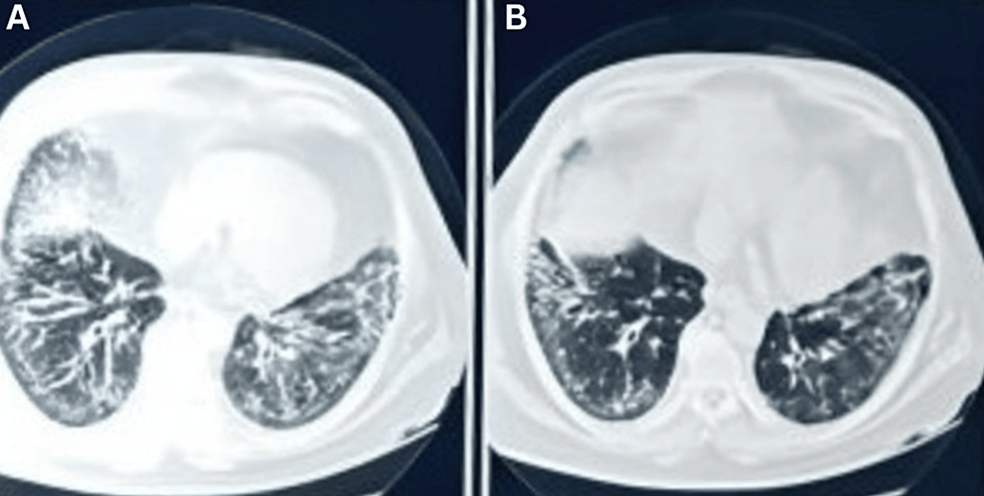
HRCT thorax A: bilateral ground glass opacities with interstitial septal thickening in bilateral lower lobes with subpleural sparing suggestive of interstitial lung disease (non-specific interstitial pneumonia pattern) and bilateral traction bronchiectasis are also seen; B: bilateral ground glass opacities in bilateral lower lobes with subpleural spacing. HRCT: high resolution computed tomograph
